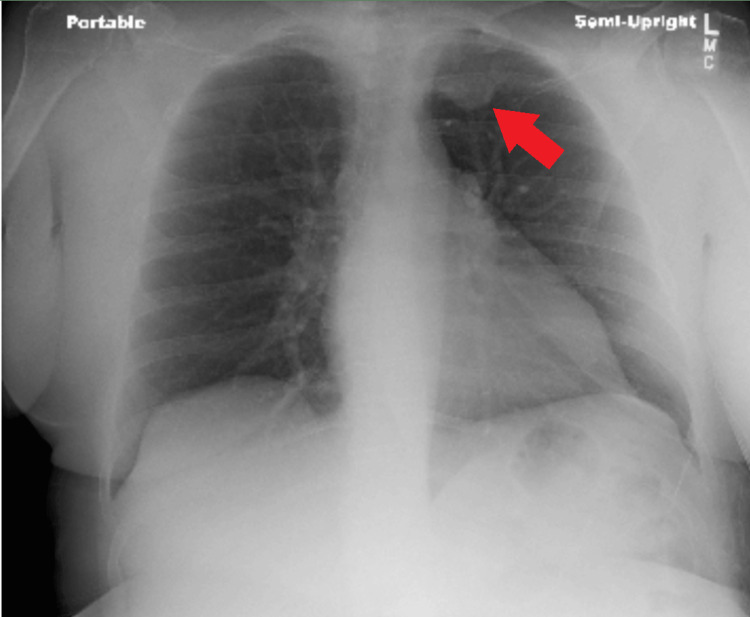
Chest radiograph demonstrating a left apical lung mass
